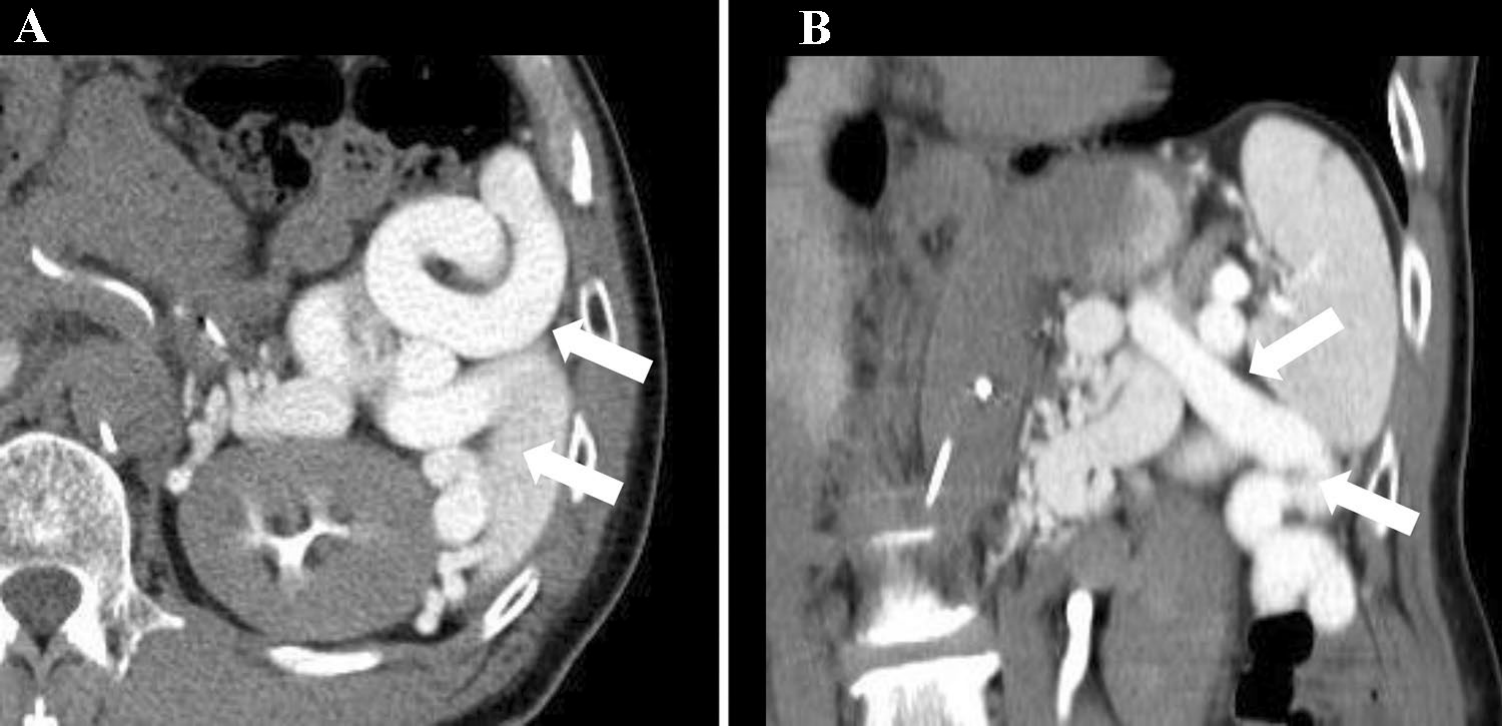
Computed tomography (CT) images showing the splenorenal shunt. a Axial CT image. b Coronal CT image. The white arrow shows the splenorenal shunt
